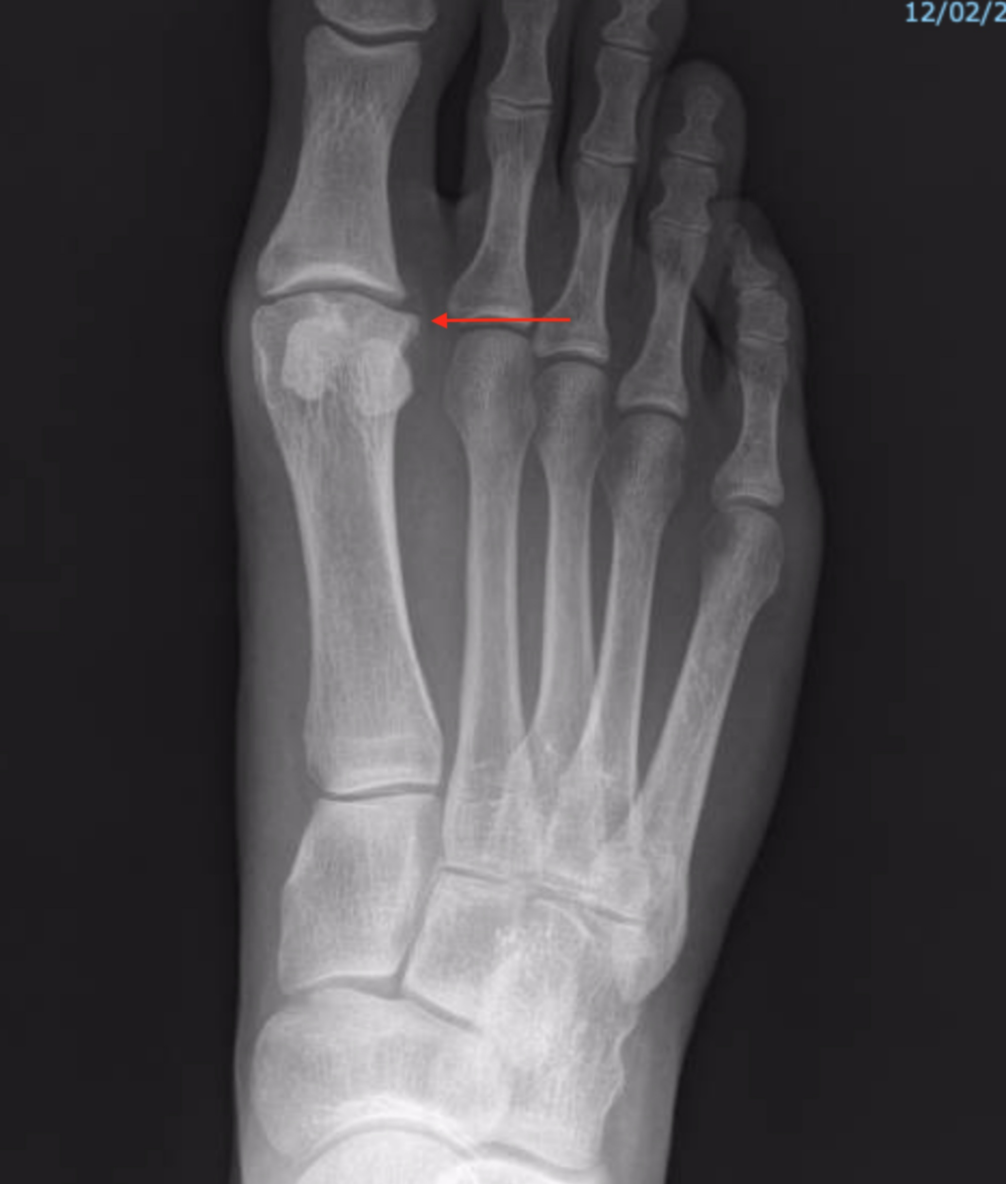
Anterior-posterior preoperative x-rays showing moderate degenerative changes of the first metatarsophalangeal joint (MTPJ).
